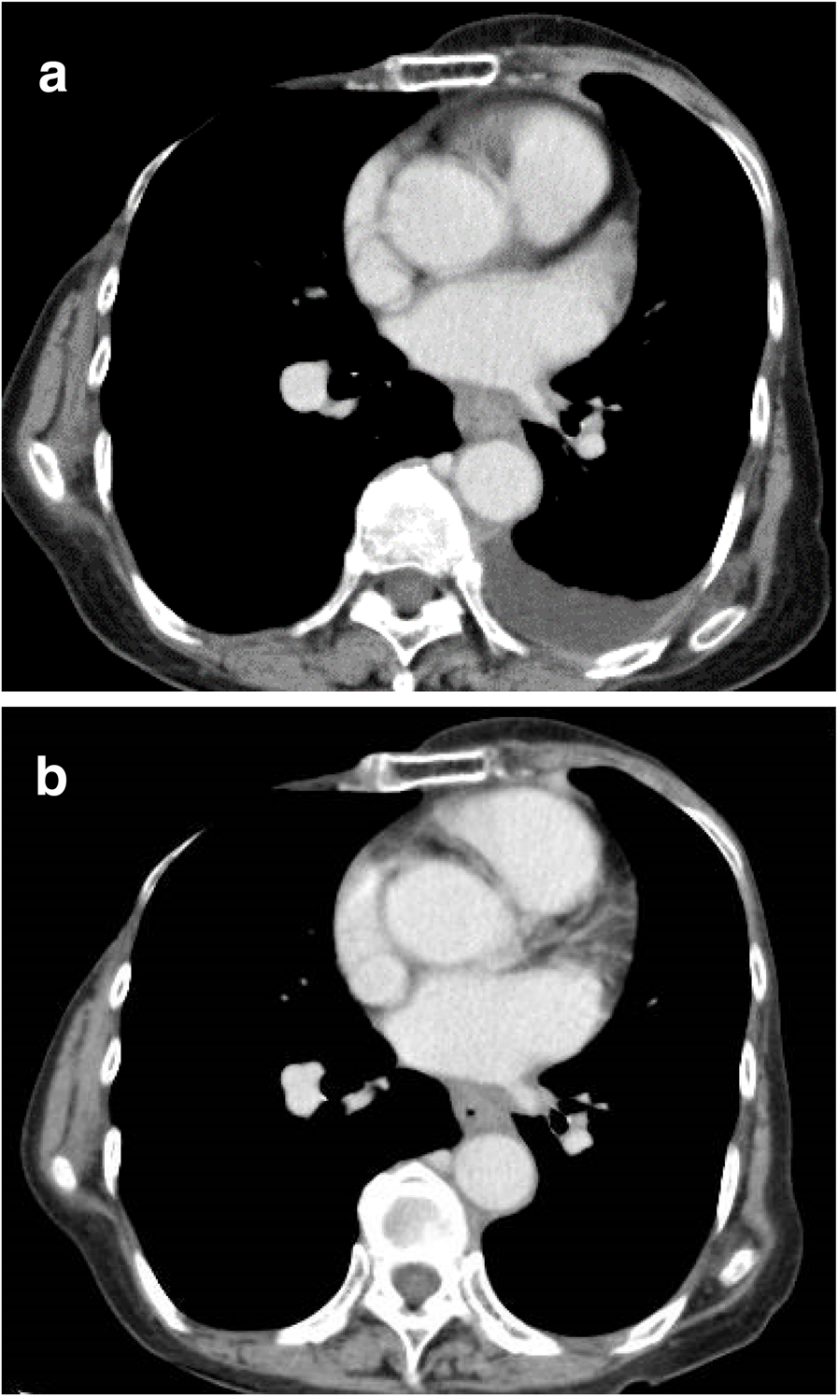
Thoracic enhanced CT findings. Thoracic enhanced CT showing wall thickening of mid-esophagus and left pleural effusion (a). After 3 months of endocrine therapy, the pleural effusion has disappeared (b)
